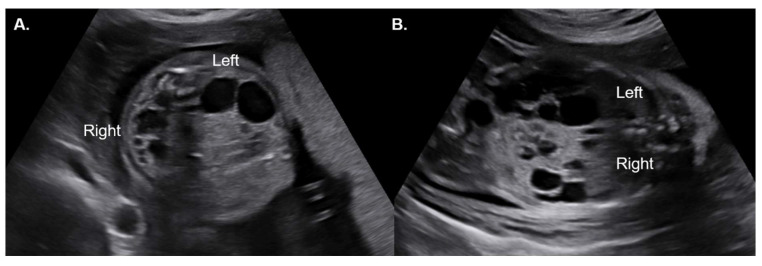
Imaging findings of a rare combined case of left MCDK and right ADPKD (Case 13). Ultrasound images demonstrating a multicystic dysplastic kidney (MCDK) on the left and ADPKD on the right. The ADPKD kidney shows multiple cysts of varying sizes, whereas the MCDK is characterized by larger, non-communicating cysts. (A) Axial plane; (B) Coronal plane.
